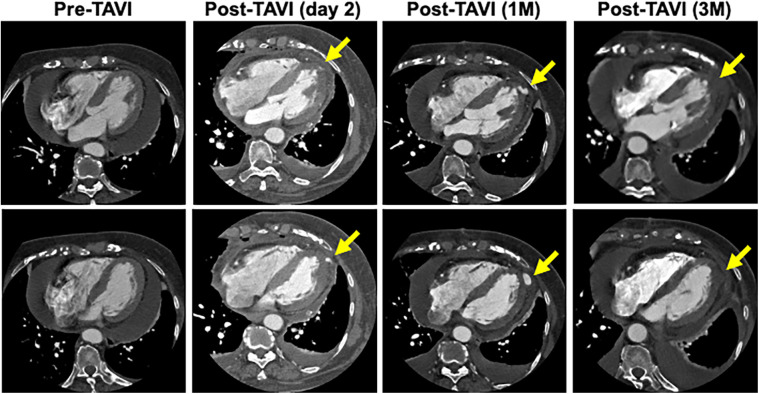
Time course of the morphology of the pseudoaneurysm. CT scan taken 3 months after repair surgery showed that the lumen of the pseudoaneurysm had disappeared.
